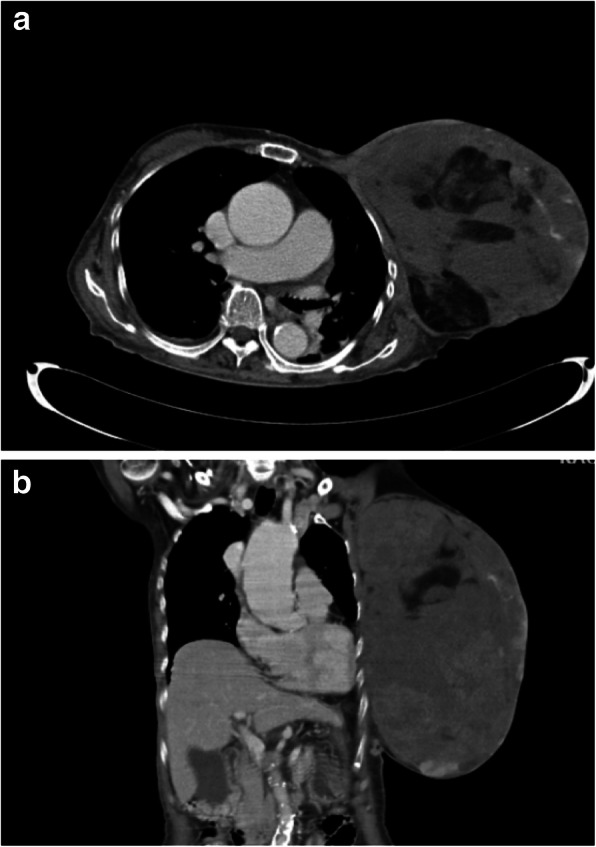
Axial view (a) and coronal view (b) of CT of chest-abdomen showed a huge breast tumor, L’t
